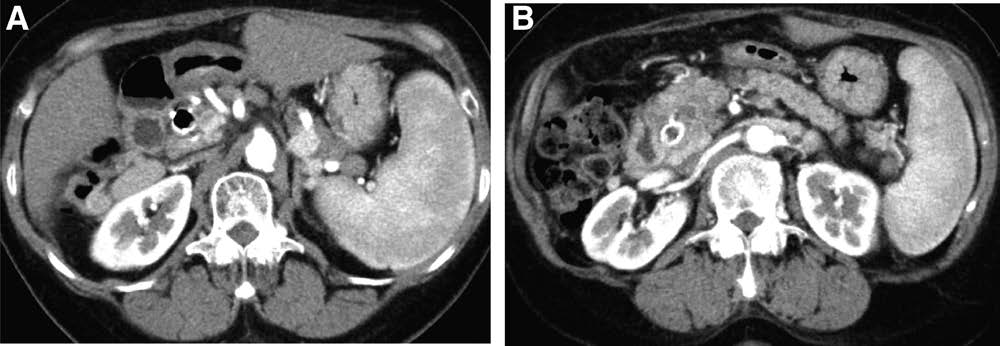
At 18 months after the diagnosis, a computed tomography scan revealed lymphadenopathies around the pancreatic body (A) but no remarkable change in the pancreatic tumor size (B).
